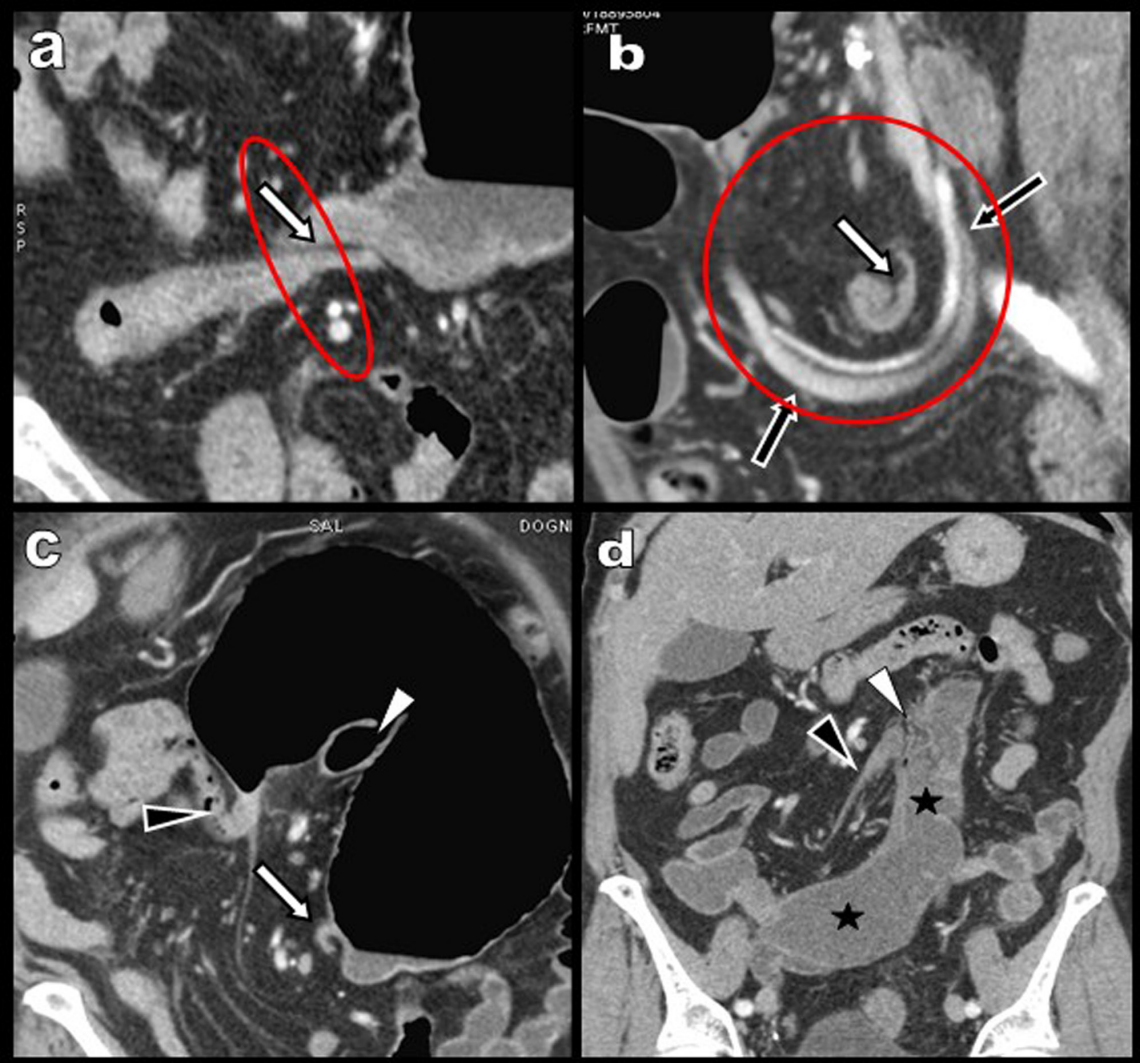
The diagnosis of cecal volvulus is confirmed surgically (Figure 3) and right hemicolectomy is performed with uneventful post-operative recovery. Interestingly, a review of the patient’s imaging data retrieves an abdominal CT performed five years earlier, already showing a wandering long ectopic cecum with neither distention nor volvulus (Figure 2d, black stars).
